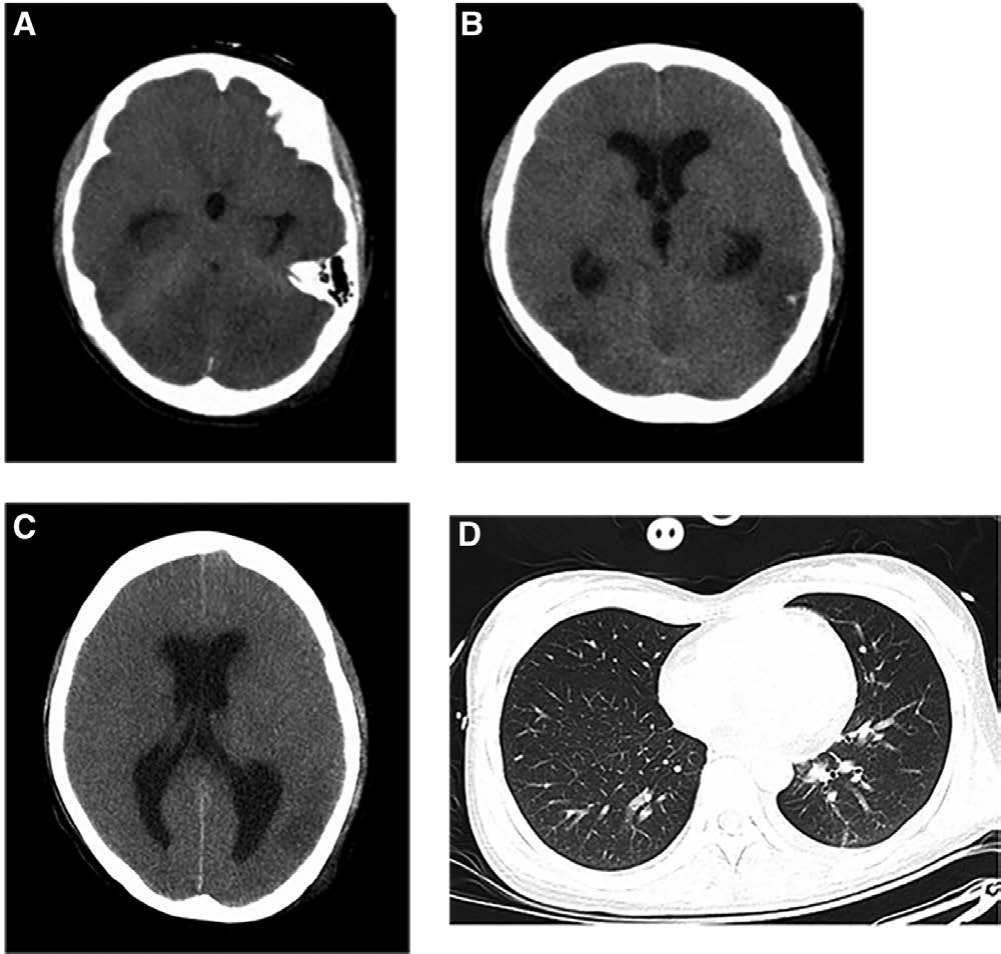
Brain and chest computed tomography imaging of the patient on day 2. Low density in bilateral cerebellar hemisphere, compression of the fourth ventricle, and interstitial cerebral edema (A), bilateral temporal occipital lobe and interstitial cerebral edema (B), lateral ventricular hydrocephalus (C); Chest computed tomography showed slight inflammation in both lower lungs (D).
